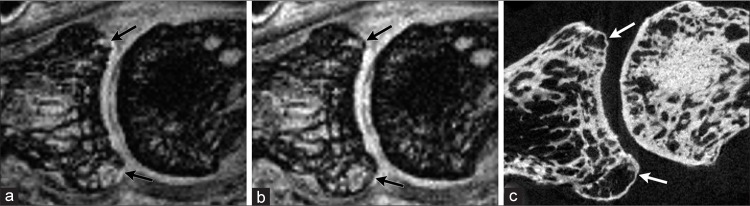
There were apparent disruptions of the marginal bone (arrows) seen on the sagittal (a) regular gradient-echo nonfat-suppressed image of this proximal interphalangeal joint but not on the corresponding (b) interleaved water-fat water + fat image. The (c) microcomputed tomography image confirmed the marginal bone was intact.
